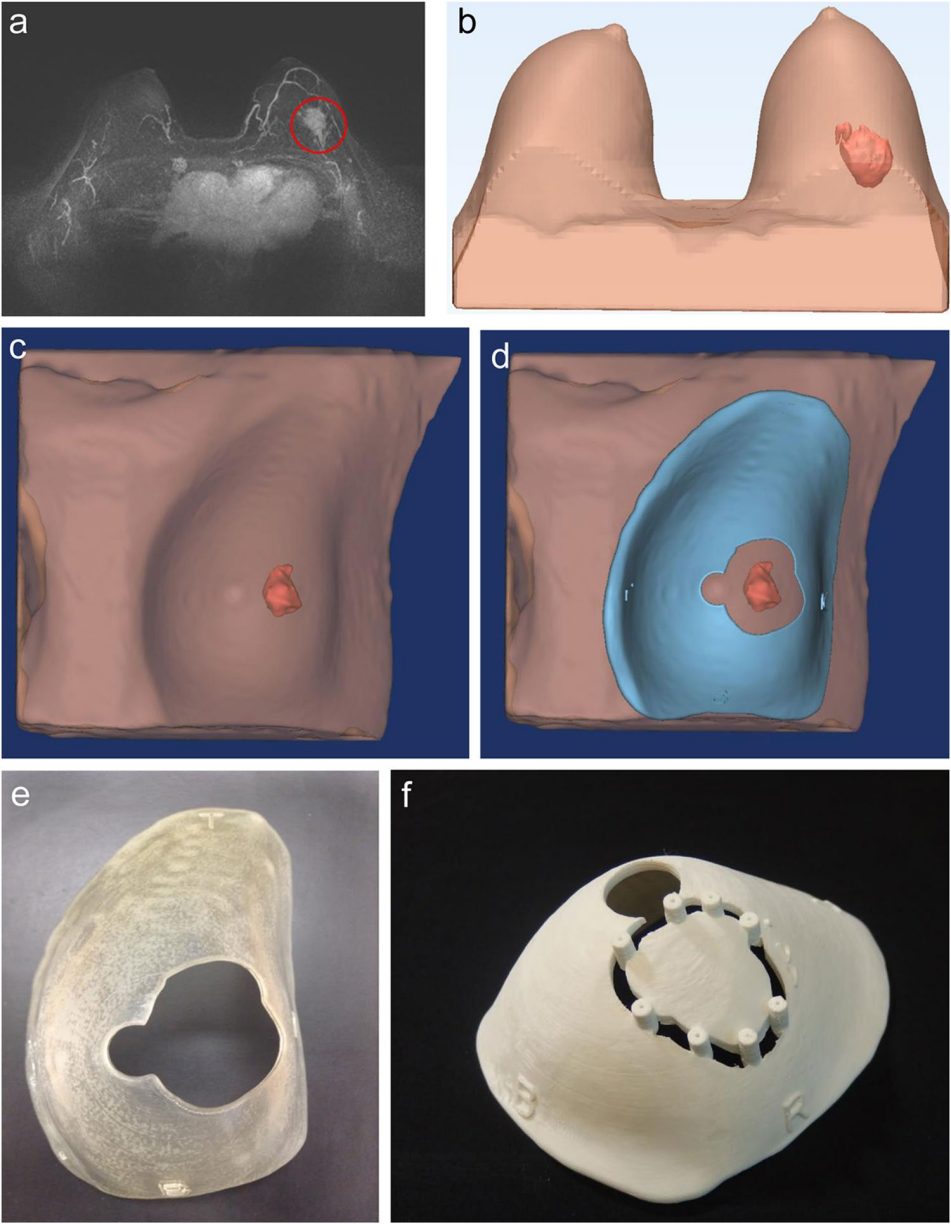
Making of 3D-printed surgical guides based on pretreatment magnetic resonance images. (a) Magnetic resonance image before neoadjuvant chemotherapy. (b) 3D printer reconstruction of breasts and tumor. (c) 3D model of the breast and tumor made prior to neoadjuvant chemotherapy. (d) Surgical guide delineating the skin surface and the extent of the tumor. (e) Skin-marking type surgical guide. (f) Hybrid type surgical guide for skin marking and dye injection.
